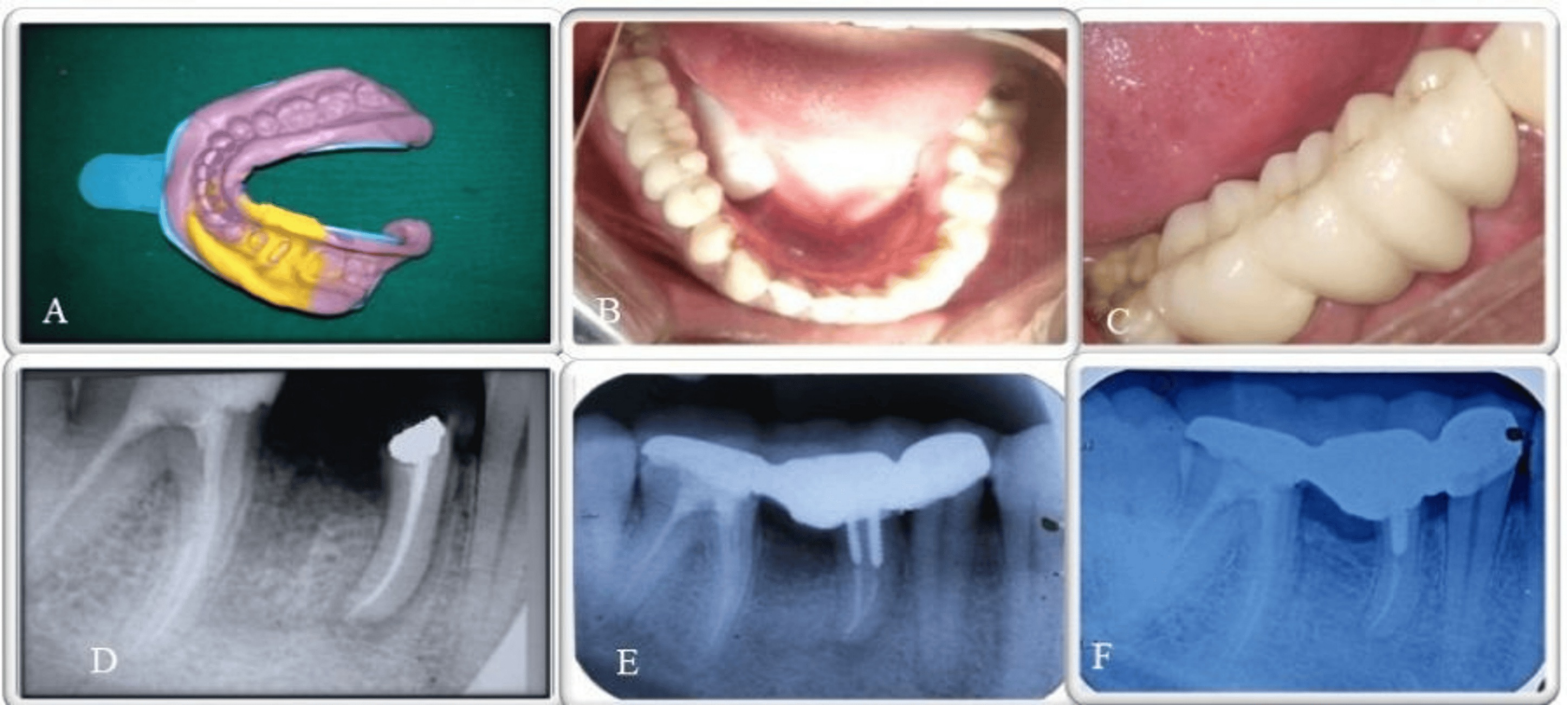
Post-operative images A) Polyvinyl siloxane impression of lower arch; B) Post-operative occlusal view of lower arch; C) Post-operative lateral view; D) One month follow-up radiograph; E) One year follow-up radiograph; F) Three-year follow-up radiograph.
